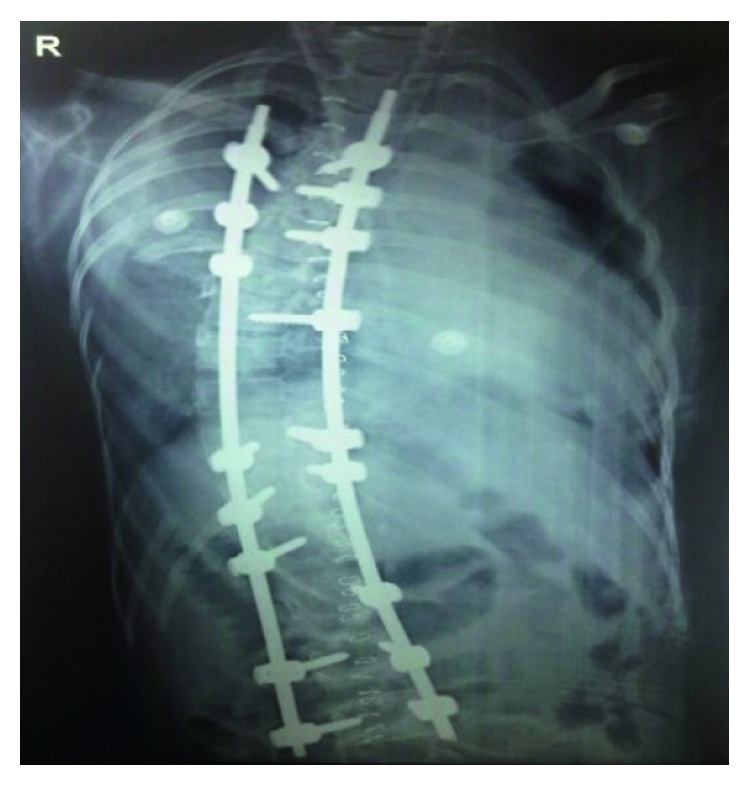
Postoperative X-ray showing correction of scoliosis with implants in situ.
